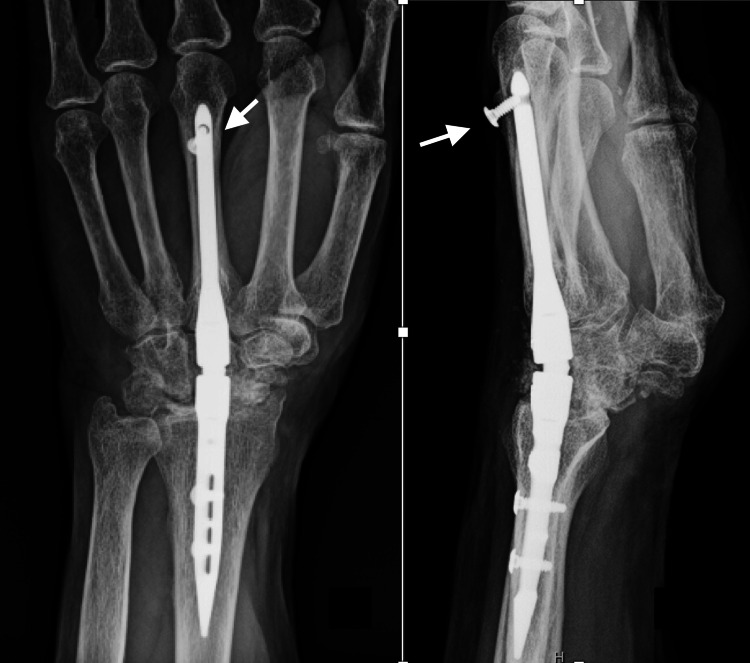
Follow-up radiographs of Case 1 Posteroanterior and lateral views at four months postop show that the distal unicortical screw backed out. The white arrows show the distal screw loosening and migration.
